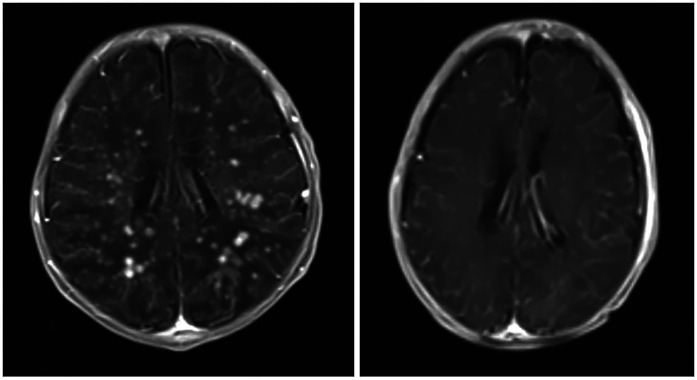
Axial views of post-contrast MRI show the difference between before (right) and after (left) treatment. The enhancing nodules have dramatically disappeared, indicating significant improvement. The previously seen meningeal enhancement is also improved, with several residual hyperintense T2 foci in the posterior periventricular region. There is also a suggestion of several tiny susceptibility-weighted imaging hypointense foci in the posterior periventricular region (sites of previous nodules), which may represent areas of calcification. This could be confirmed by a follow-up brain CT.
